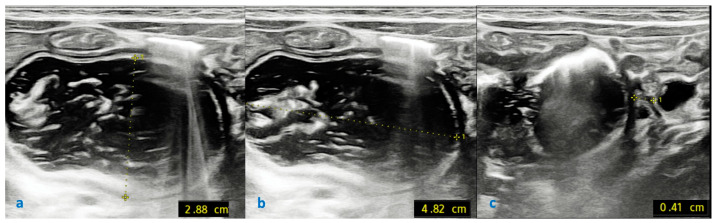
Ultrasound: dilated duodenum with transverse diameter of 2.88 cm (a) and longitudinal diameter of 4.82 cm (b); intraluminal diaphragm with central aperture of 0.41 cm at site of obstruction (c).
